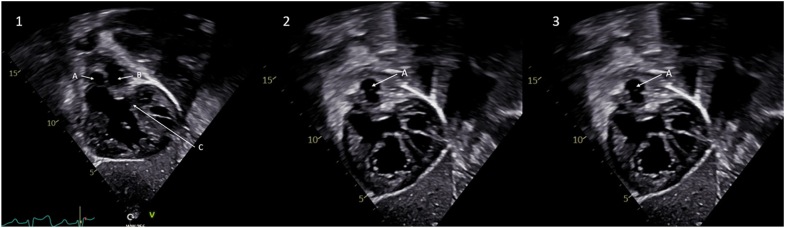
Preoperative echocardiography shows the (1) aorta (A), pulmonary artery (B), and ventricular septal defect (VSD) (C); (2) the inlet VSD (A) and (3) the pulmonary artery (A) far away from the VSD.
